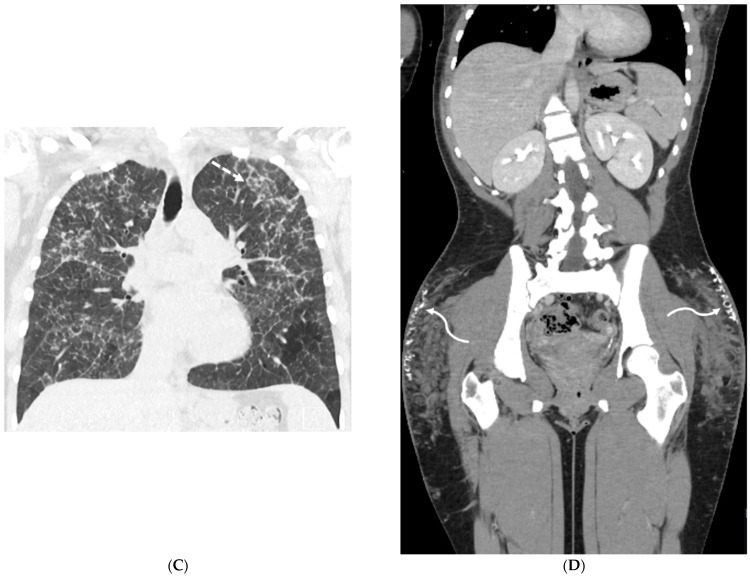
A 24-year-old female with a history of silicone injection of the buttocks presents with shortness of breath. A frontal chest radiograph (A) shows diffuse interstitial thickening. Axial (B) and coronal (C) chest CT images demonstrate upper- and mid-lung-zone-predominant perilymphatic nodularity (solid white arrow), interlobular (dashed white arrows) and intralobular nodular septal thickening, and irregular nodules associated with areas of interstitial thickening. A coronal pelvis CT image (D) demonstrates infiltrative soft tissue granulomas (curved white arrows), some calcified, with admixed areas of fat necrosis in bilateral gluteal subcutaneous fat.
